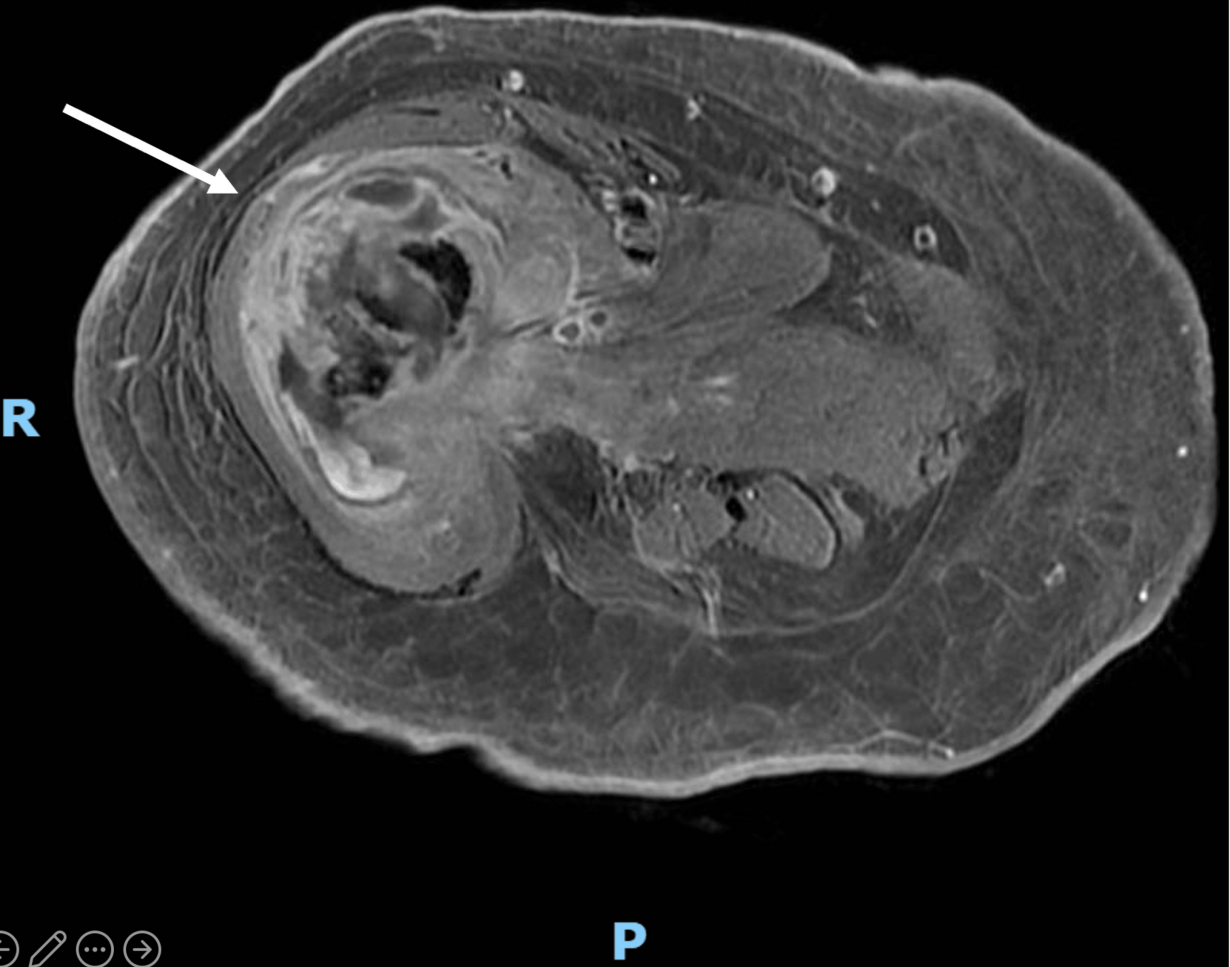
MRI of the right thigh showing multiloculated multiseptated collection (arrow) with peripheral enhancement at fracture site measuring 4.4 x 3.4 cm
